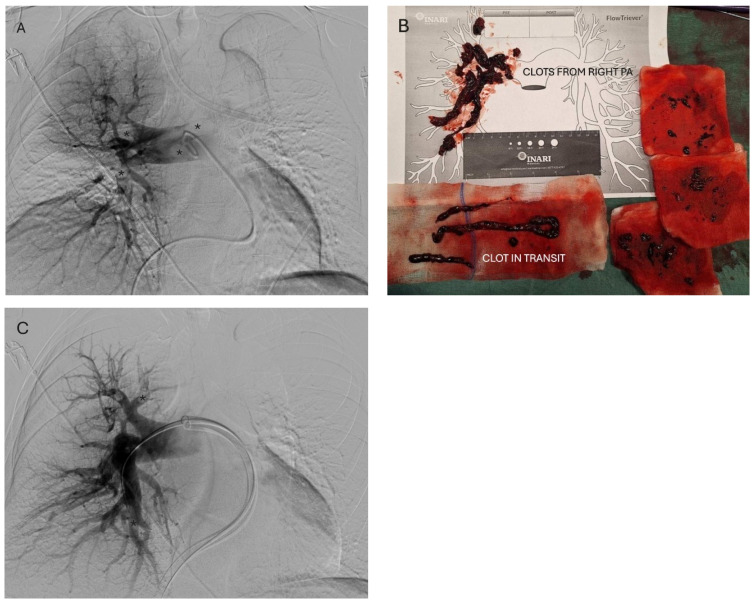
(A) Catheter angiography shows contrast filling defects (annotated with stars, *) in the right pulmonary artery branches. According to the 2019 guidelines [6], systemic thrombolysis is the recommended treatment for patients in obstructive shock. However, contraindications to systemic thrombolysis are common in high-risk PE patients, particularly in the postoperative setting. Although these guidelines do not provide recommendations for the treatment of high-risk PE patients with CIT, a recent study suggests that in patients with CIT, systemic thrombolysis or catheter-based treatment, including mechanical thrombectomy, is associated with a significantly lower rate of adverse clinical outcomes compared to anticoagulation alone [7]. Given the patient’s recent abdominal surgery and haemodynamic instability, systemic thrombolysis was contraindicated. After a multidisciplinary team discussion, the decision was made to manage CIT and PE with mechanical thrombectomy, which offers the possibility of rapid reduction in right ventricular strain without added bleeding risk [8]. Under local anesthesia and ultrasound-guided navigation, left common femoral vein puncture was performed, followed by over-the-wire placement of a 24 French introducer sheath (Intri 24, Inari, Irvine, CA, USA). A large-bore aspiration catheter (FlowTriever 24, Inari, Irvine, CA, USA) was advanced over the stiff wire (Amplatz Super Stiff, Boston Scientific, Marlborough, MA, USA) and navigated to the right atrium, where a single aspiration with a dedicated syringe was performed, resulting in incomplete CIT removal. The same aspiration catheter was then navigated to the right pulmonary artery, where six aspirations were performed, evacuating the majority of the right-sided pulmonary thrombus (B). Control angiography demonstrated improved filling of the right pulmonary artery branches with only small residual thrombi (C). During catheterization of the left pulmonary artery, the patient experienced ventricular tachycardia due to wire manipulation. As the patient’s vital parameters improved—blood pressure 110/60 mmHg, pulse 75 beats per minute, and SpO_2_ 99%—further attempts at thrombectomy were deemed unnecessary. The puncture site was closed with manual compression assisted by a haemostatic sponge. Repeat echocardiography showed normal right atrial and ventricular dimensions, full recovery of right ventricular systolic function (TAPSE 22 mm), and no visible thrombus in the right atrium or pulmonary artery. Throughout the hospital stay, the patient remained haemodynamically stable with no further intervention required.
